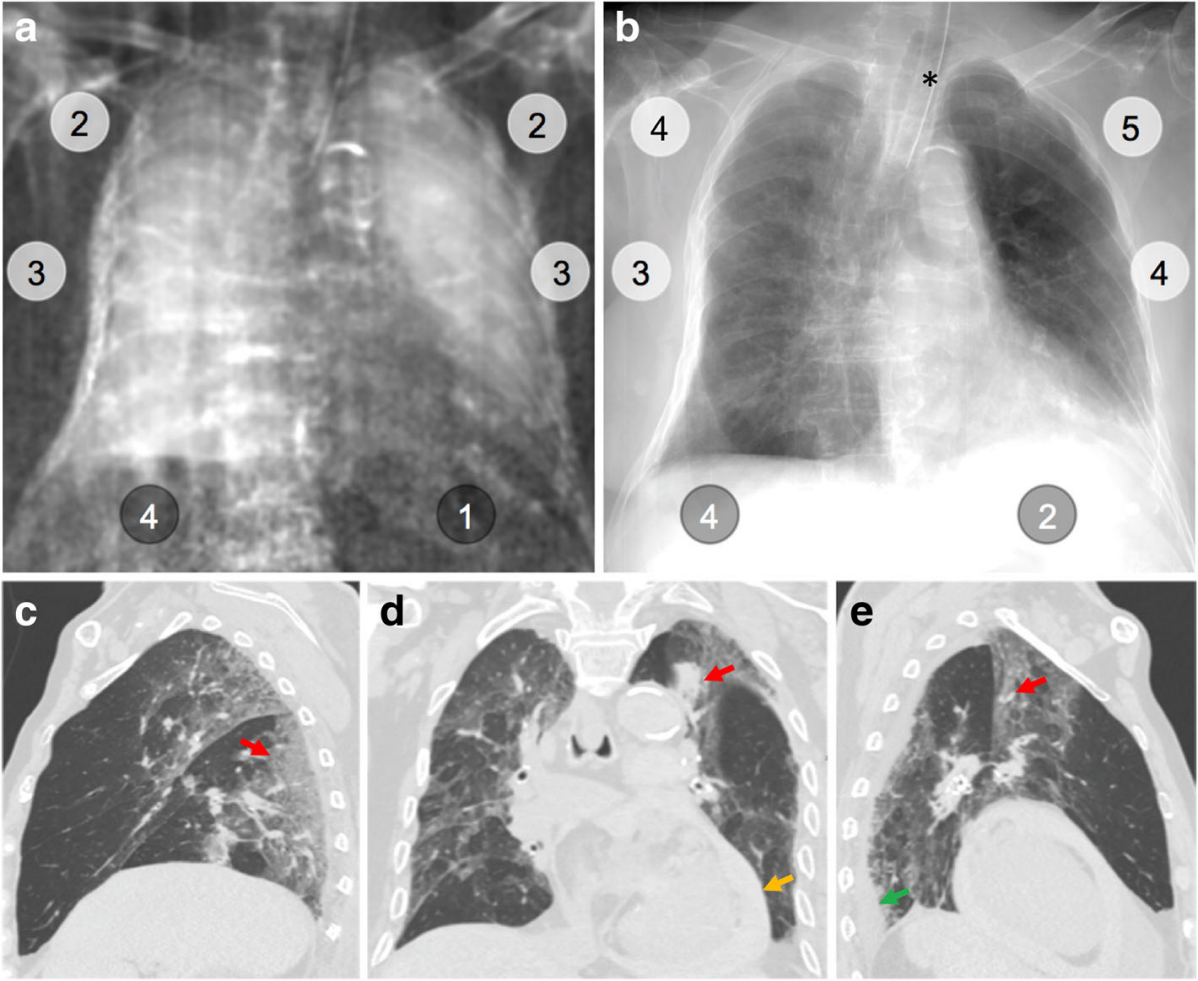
Dark-field chest radiograph, conventional x-ray, and CT of human body 8. Median visual grading (encircled numbers) of dark-field chest radiograph (a) by three independent readers shows a markedly reduced dark-field signal in left lung lower zone and to a minor degree in both middle zones, compared to other regions. Median visual grading of transmission in conventional x-ray (b) shows reduced transmission in the right lung, especially in the middle zone, and a markedly reduced transmission in the lower zone of the left lung. Sagittal (c, e) and coronal (d) CT images show interstitial and alveolar infiltrates (red arrows) in depending parts of the right and left upper and lower lobes. Furthermore, there is an enlargement of the heart with a significant haemopericardium (orange arrow) and small left-sided pleural effusion (green arrow). Asterisk marks the location of the endotracheal tube
